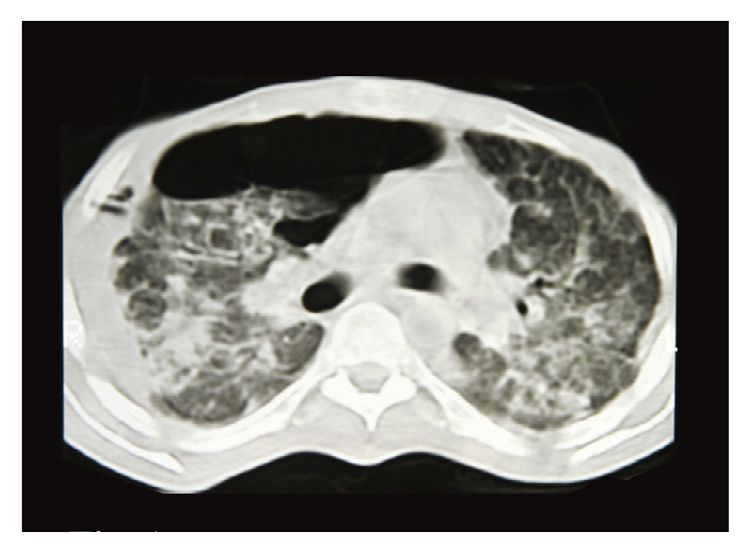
CT. 1: Large localized pneumothorax in right lung. 2: Mixed alveolar and interstitial fibrosis. 3: Pleural thickening in right lung. 4: Several bullae in right lung. 5: Alveolar and interstitial shadowing. 6: Paraseptal emphysema in anterior segment of left upper lobe.
